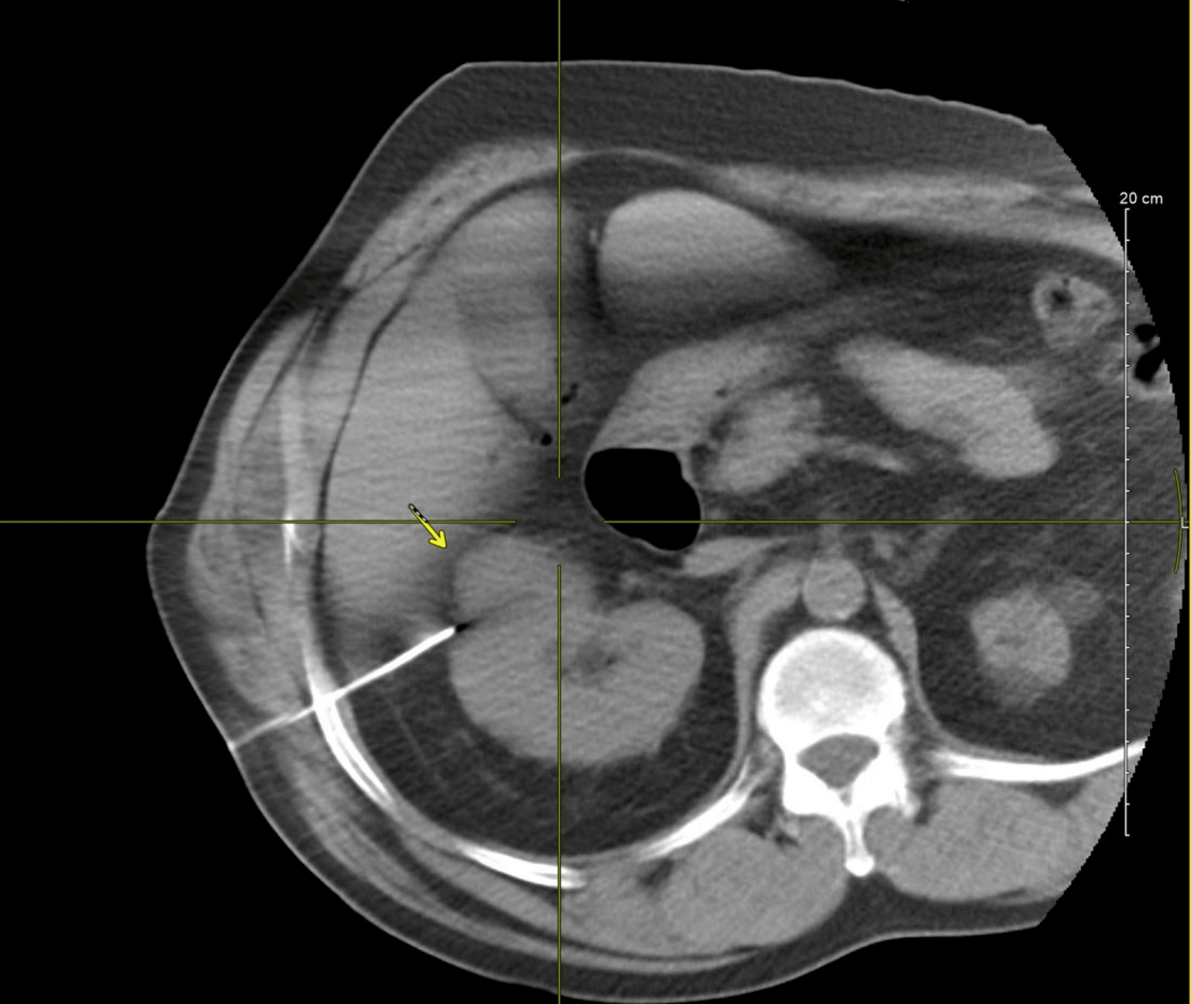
Axial, IR-guided thermal ablation Using ultrasound guidance, a 16-gauge guiding needle was advanced to 3 cm from the margin of the renal mass, and microwave ablation was performed at 60W for 15 minutes with no immediate hemorrhage or other complications. Extensive hydrodissection was done prior to ablation, performed using the guiding needle to separate the colon from the kidney, while approximately 150 cc of 1% contrast in the 5W was injected to separate the colon from the kidney. IR: interventional radiology
